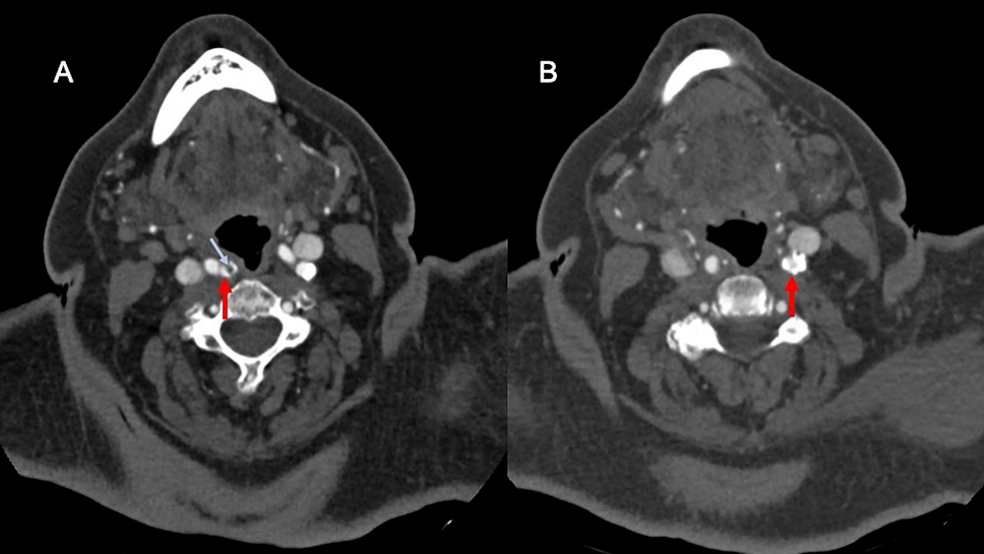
Axial contrast-enhanced CT images of the neck (A, B) demonstrate moderate stenosis of the proximal ICAs, as per NASCET criteria (red arrows in A and B). Additionally, there is a 5.6 mm ulcerated atheromatous plaque in the proximal right ICA (blue arrow in A). ICA, internal carotid artery
